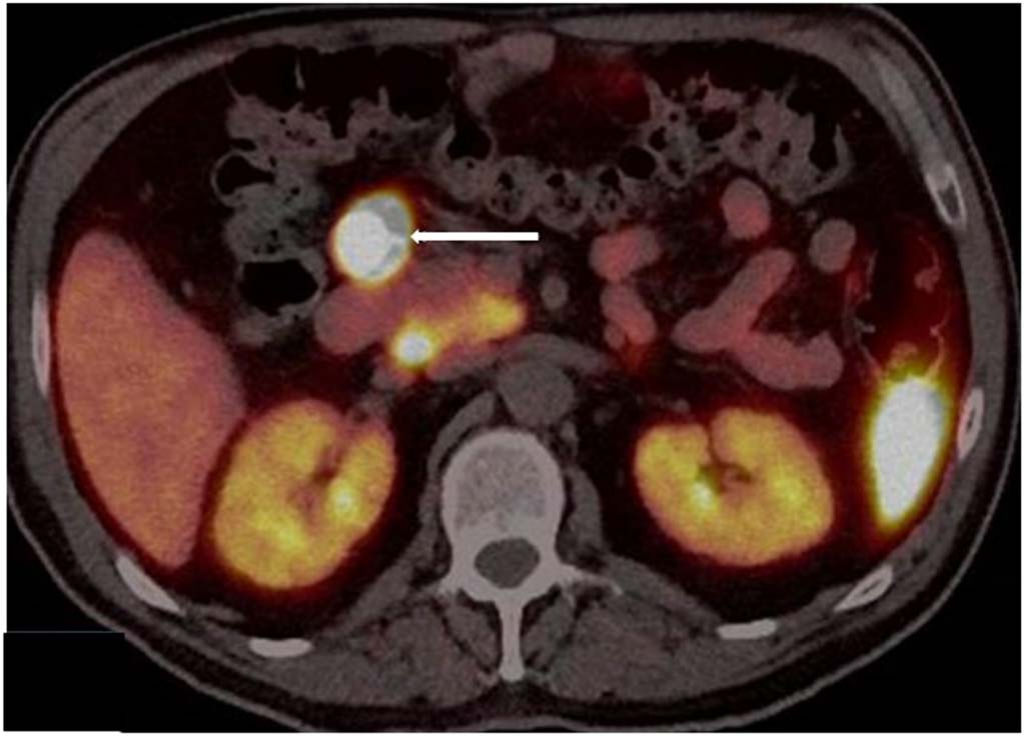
Ga-68 DOTATOC positron emission tomography/computed tomography scan showing somatostatin receptor–positive peripancreatic node (arrow).
